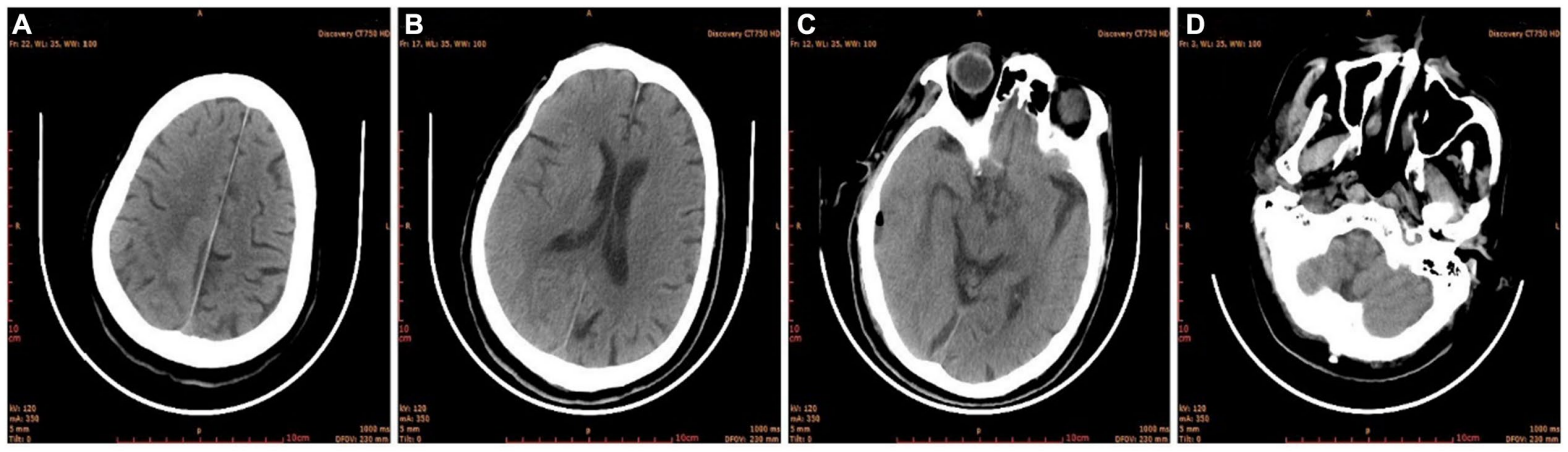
Brain CT before discharge. (A) Semi-oval central level; (B) lateral ventricular body level; (C) cerebral base level; (D) skull base level.
